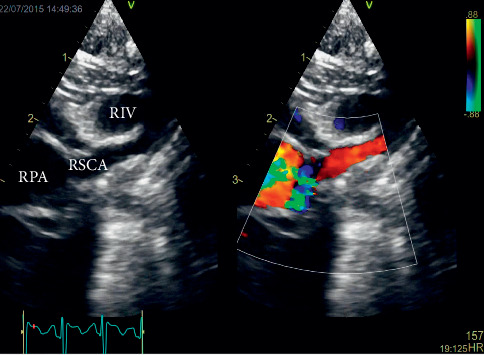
Echocardiography of the newborn with B-image on the left and a simultaneous color Doppler on the right; in a high right parasternal long axis, an aberrant origin of the right subclavian artery (RSCA) from the right pulmonary artery (RPA) could be displayed with course towards the right arm. RIV, right innominate artery.
